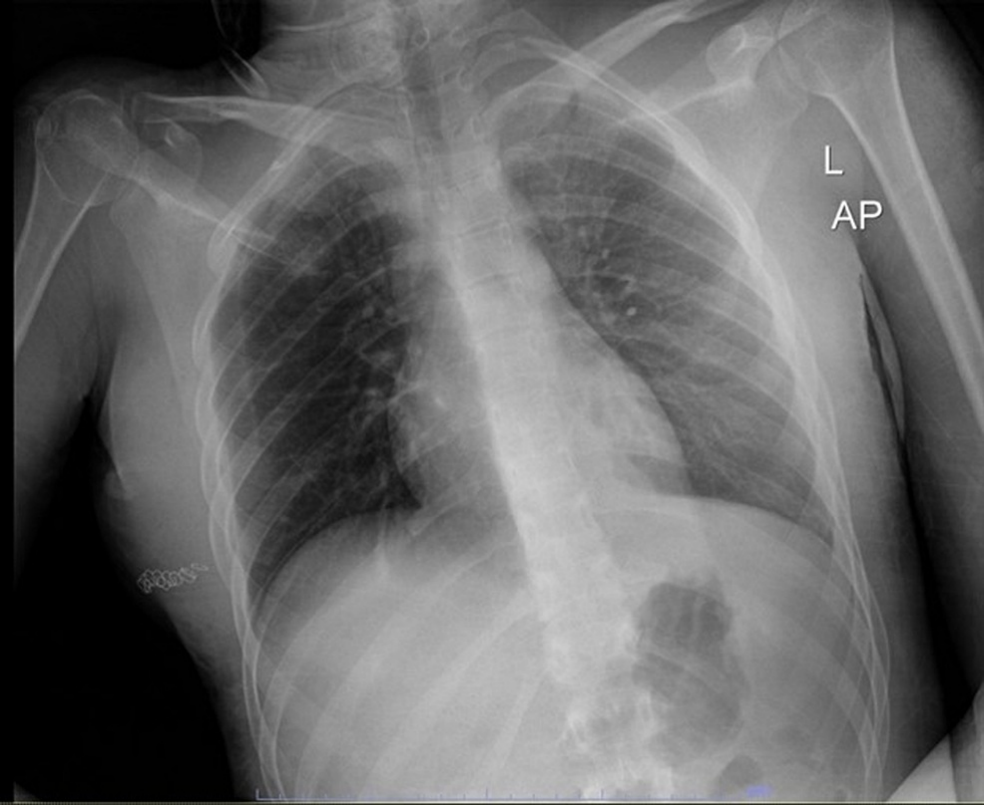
X-ray of the chest three days post-trauma. Note bilateral first rib fractures.
